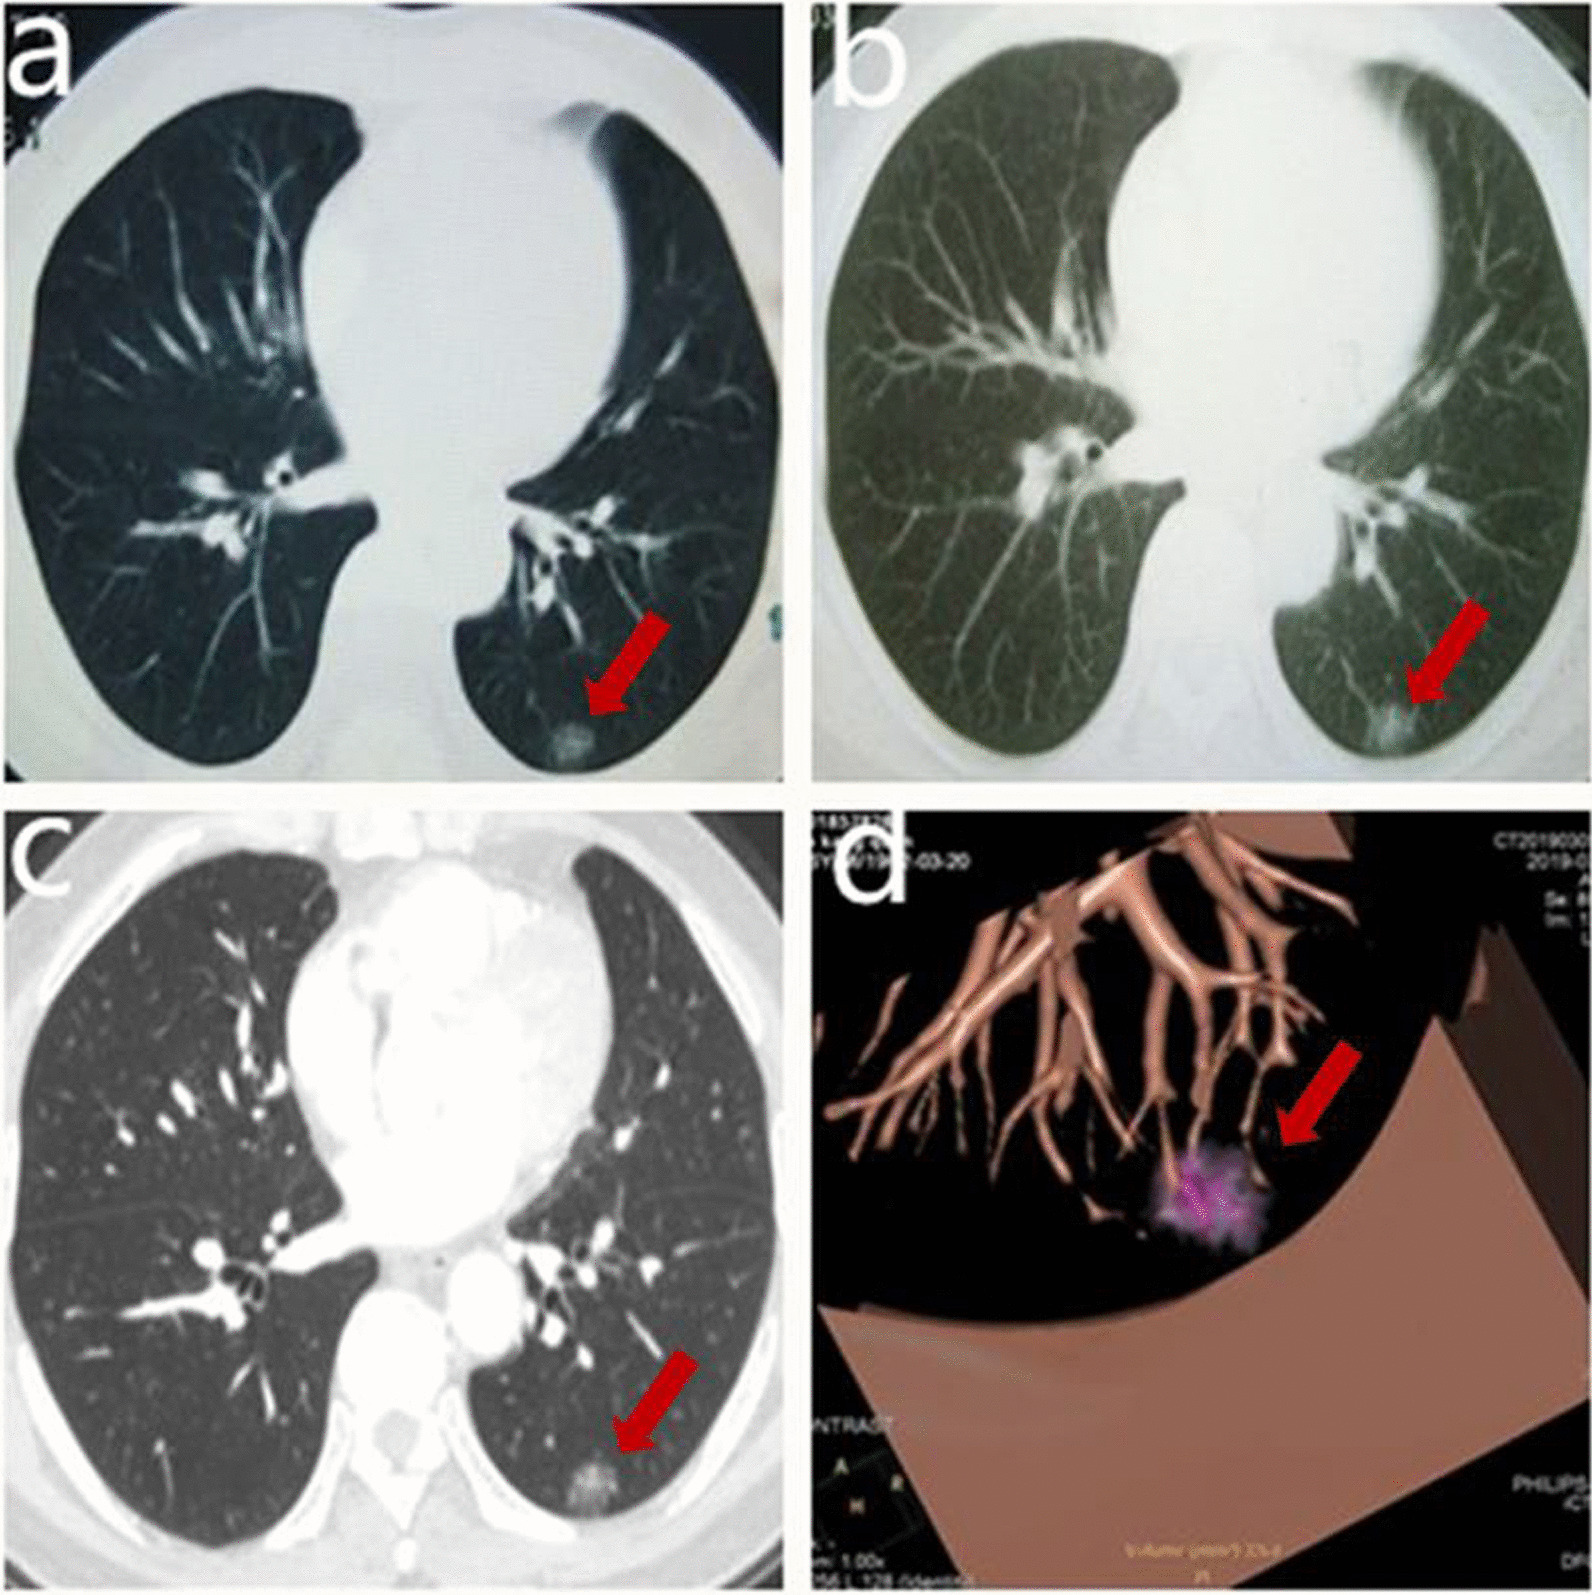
The CT scan of the patient. a Initial CT scan performed in July 2017. b Follow-up CT scan performed in November 2018. c Follow-up CT scan performed March 2019. d The three-dimensional reconstruction of CT scan performed in March 2019
